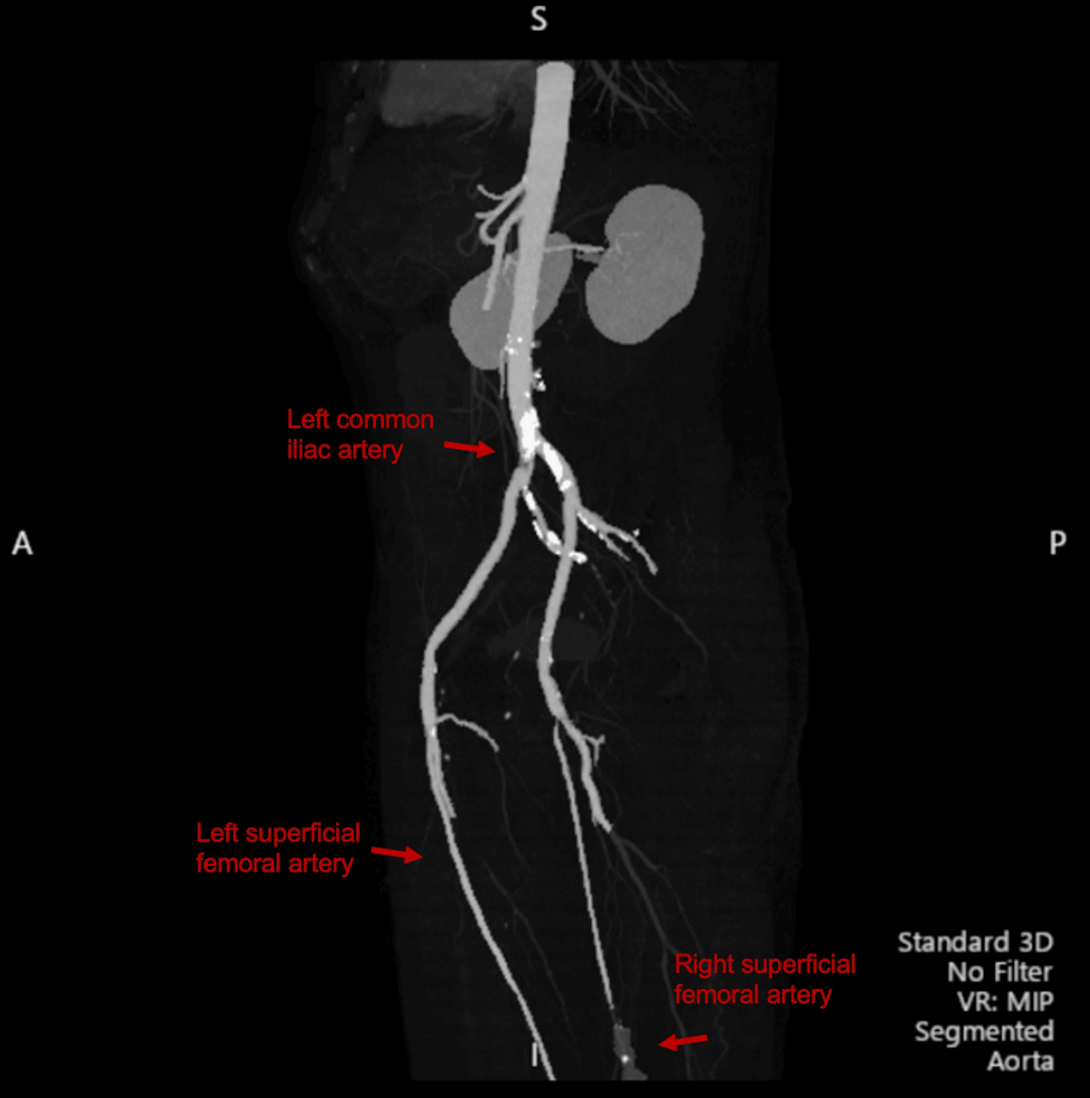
CT-angiography findings. The lower extremities revealed a large acute on chronic thrombus in the distal left common iliac artery. A long segment of complete occlusion of the distal right superficial femoral artery with reconstitution via collaterals supplied by the profunda as it entered the adductor hiatus with distal perfusion.
